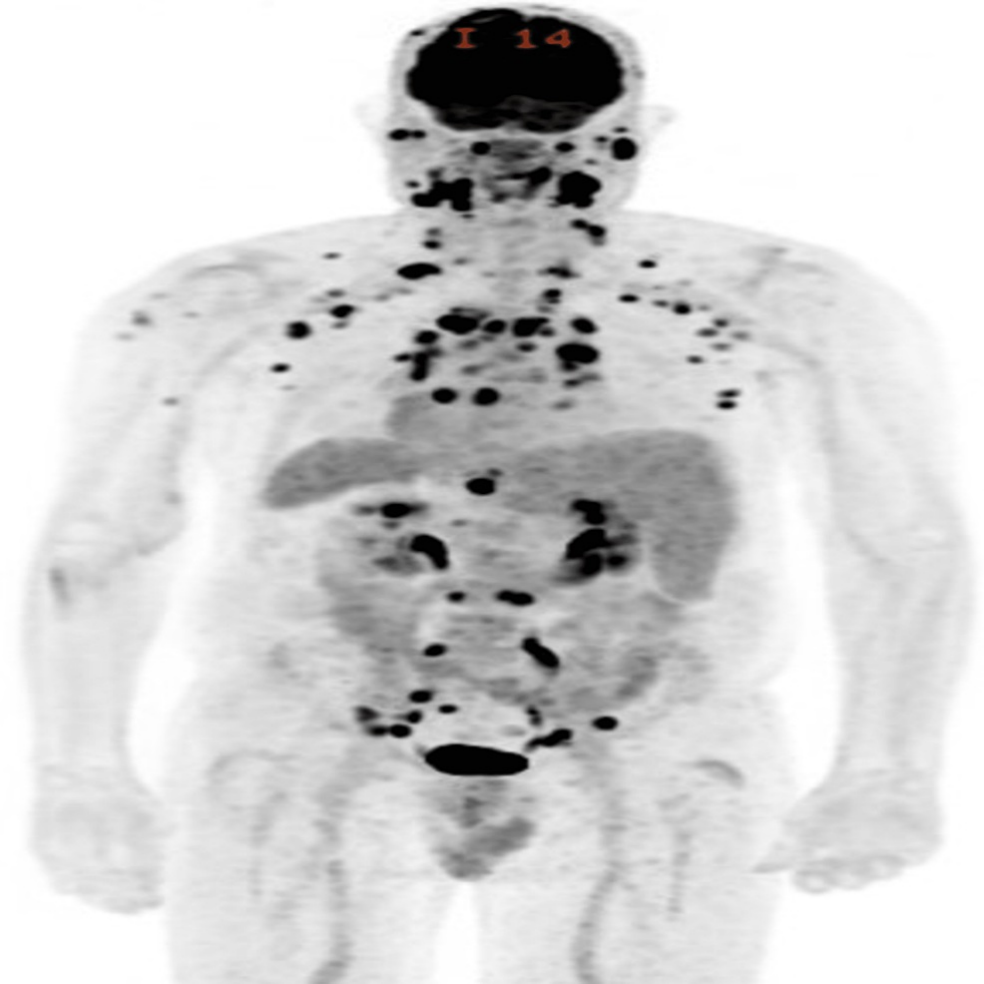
PET scan of the patient with biopsy-confirmed BPDCN after failure of two lines of therapy showing multiple FDG-avid sites of disease PET: Positron emission tomography; BPDCN: blastic plasmacytoid dendritic cell neoplasm; FDG: F-fluorodeoxyglucose
